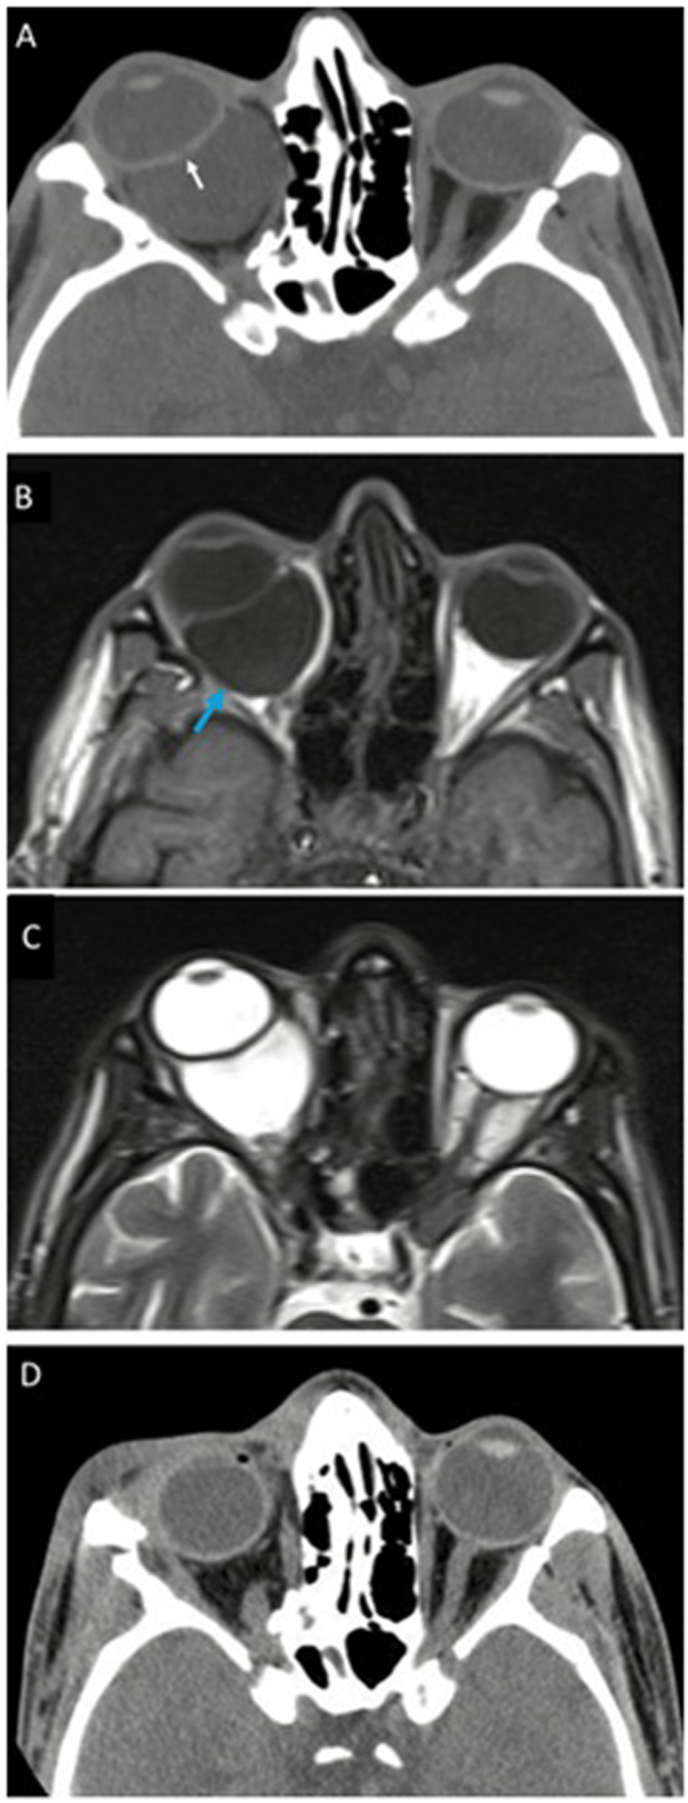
(A) Axial orbital computed tomography (CT) showing proptosis of the right eye with fractures of the lateral and medial orbital walls, and a retrobulbar cyst and the full-thickness retinochoroidoscleral defect (white arrow). (B) Magnetic resonance imaging (MRI) demonstrates a well-defined retrobulbar cystic lesion posterior to the globe, which was hypointense on T1-weighted sequences without contrast enhancement (blue arrow) and (C) hyperintense on T2-weighted images. (D) CT performed 4 weeks after surgical removal of orbital cyst. (For interpretation of the references to colour in this figure legend, the reader is referred to the Web version of this article.)
